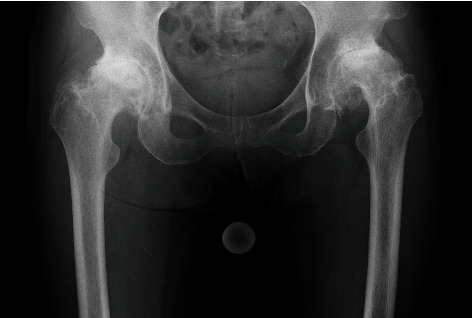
The plain radiograph before surgery. End-stage osteoarthritis was revealed in both hips.
